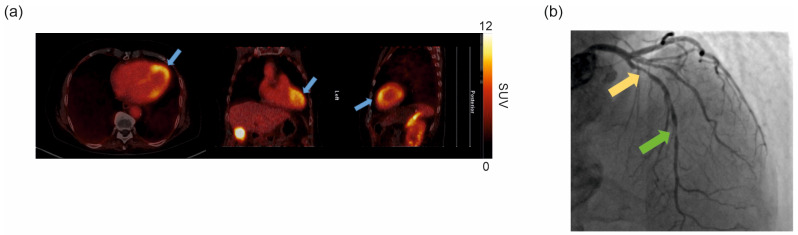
Representative clinical cardiac images: PET/CT images performed 120 min after i.v. tracer injection. Enhanced [^68^Ga]Ga-DATA^5m^.SA.FAPi uptake is observed in the mid-distal anterior LV segments in axial (left), coronal (middle), and sagittal (right) views (blue arrows) (a). Coronary angiography image: Right anterior oblique cranial view shows the left coronary artery system with a significant stenosis on the proximal LAD coronary artery (yellow arrow). A further significant stenosis with subtotal occlusion can be observed on the mid-LAD coronary artery (green arrow) (b).
